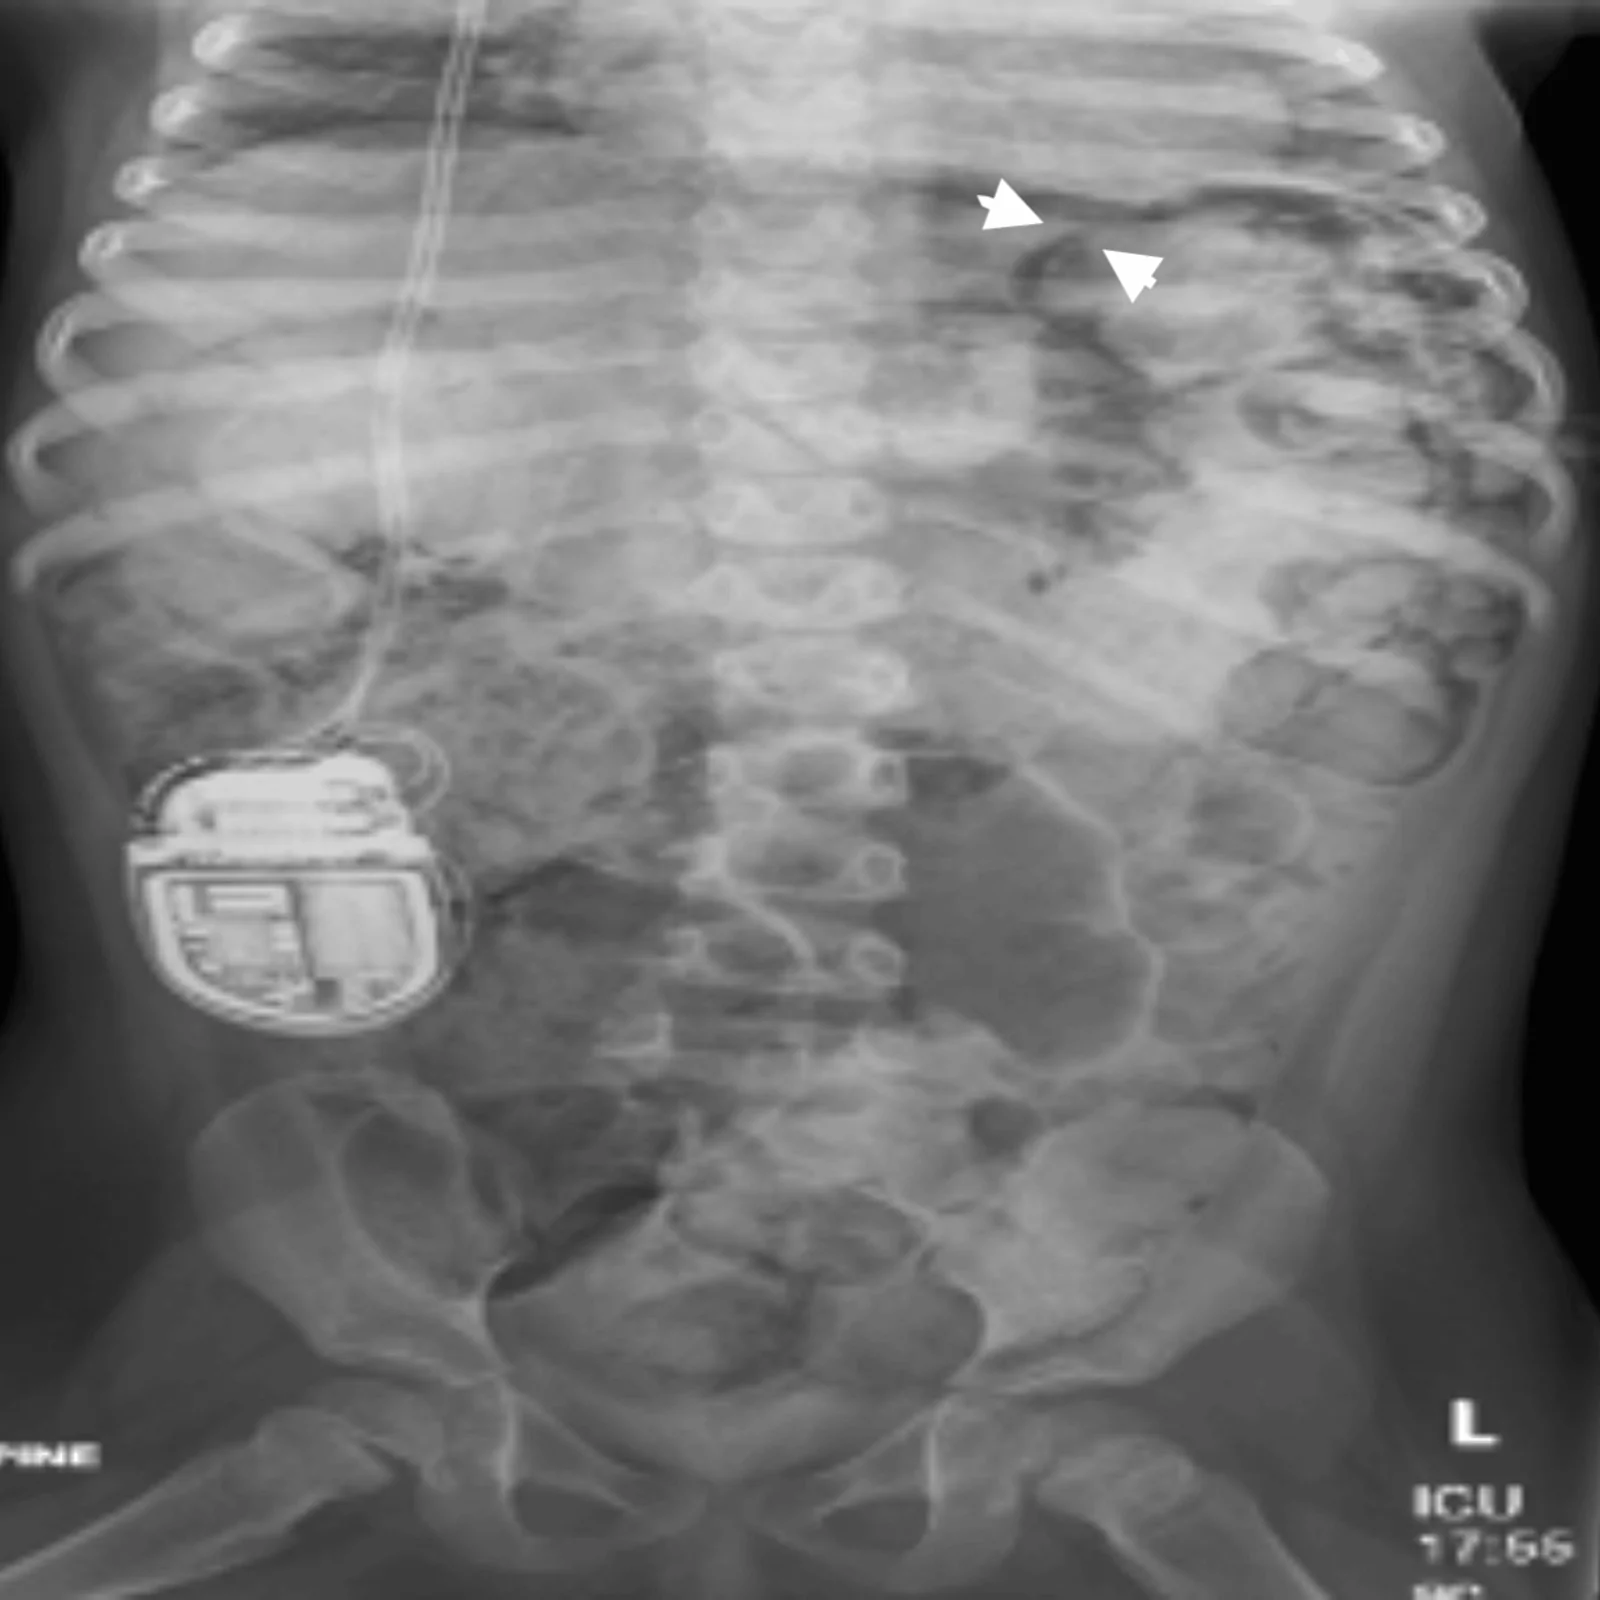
X-ray abdomen Day 2 post admission Positive Rigler sign (arrowheads) with evidence of pneumoperitoneum from extraluminal gas demonstrating a double-lumen pattern. Considerable amount of stool in the right and transverse colon. A deep-brain stimulator is demonstrated in the soft tissues of the right iliac fossa.
